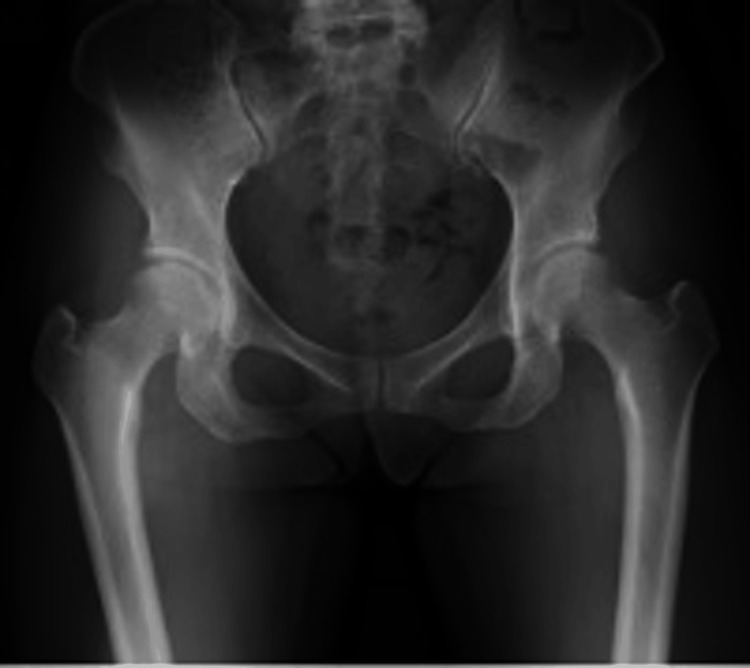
Preoperative radiographs of case 1. CE angle: right 15.4°, left 19.1°; ARO: right 17.8°, left 15.2°; Sharp's angle: right 42.5°, left 40.4°; AHI: right 72.5%, left 72.5%. CE, center–edge; ARO, acetabular roof obliquity; AHI, acetabular head index
